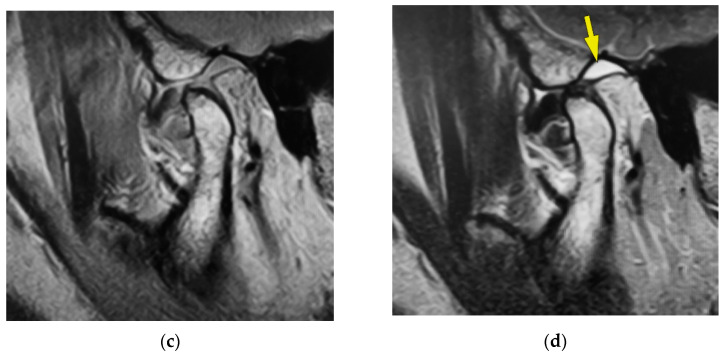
MR image of one patient with disc displacement without reduction. (a) Proton density-weighted sagittal oblique cross-section imaging at the closed mouth position. (b) T2-weighted sagittal oblique cross-section imaging at the closed mouth position. The yellow arrow indicates the area of joint effusion. (c) Proton density-weighted sagittal oblique cross-section imaging in the maximum mouth-opening position. (d) T2-weighted sagittal oblique cross-section imaging in the maximum mouth-opening position. The yellow arrow indicates the area of joint effusion.
